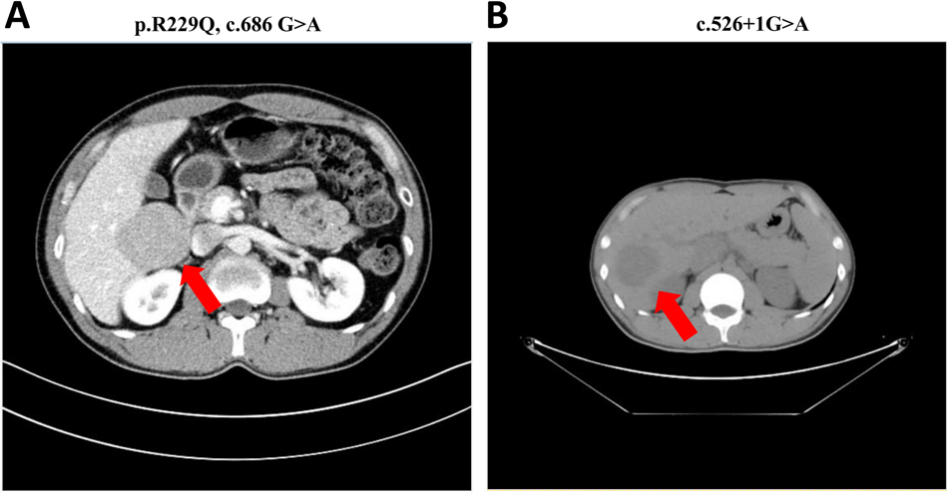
Computed tomography (CT) scans of the two probands. CT scans taken on the preoperative day (a: proband 1; b: proband 2). The size of the tumors of proband 1 was 60 mm × 57 mm × 48 mm (52 HU), and that of proband 2 was 47 mm × 42 mm (48 HU) (arrowhead)
